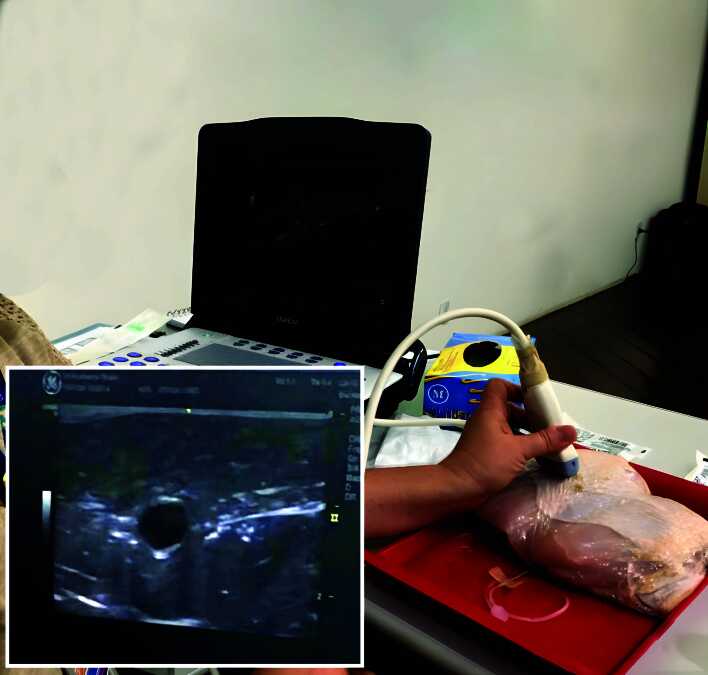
Ultrasonographic image of the puncture model. Source: authors’ files.
